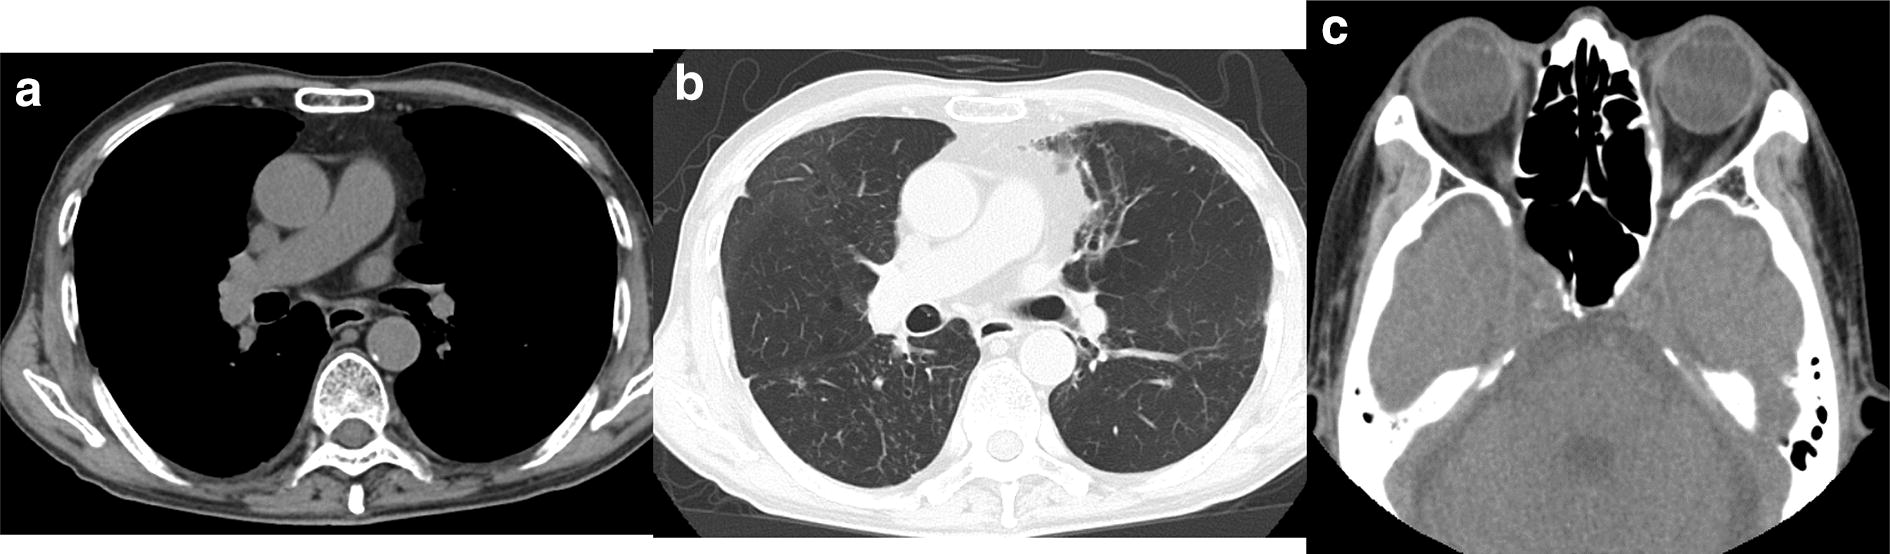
a–c Computed tomography scan of the lung and paranasal sinuses showing apparent amelioration with treatment
